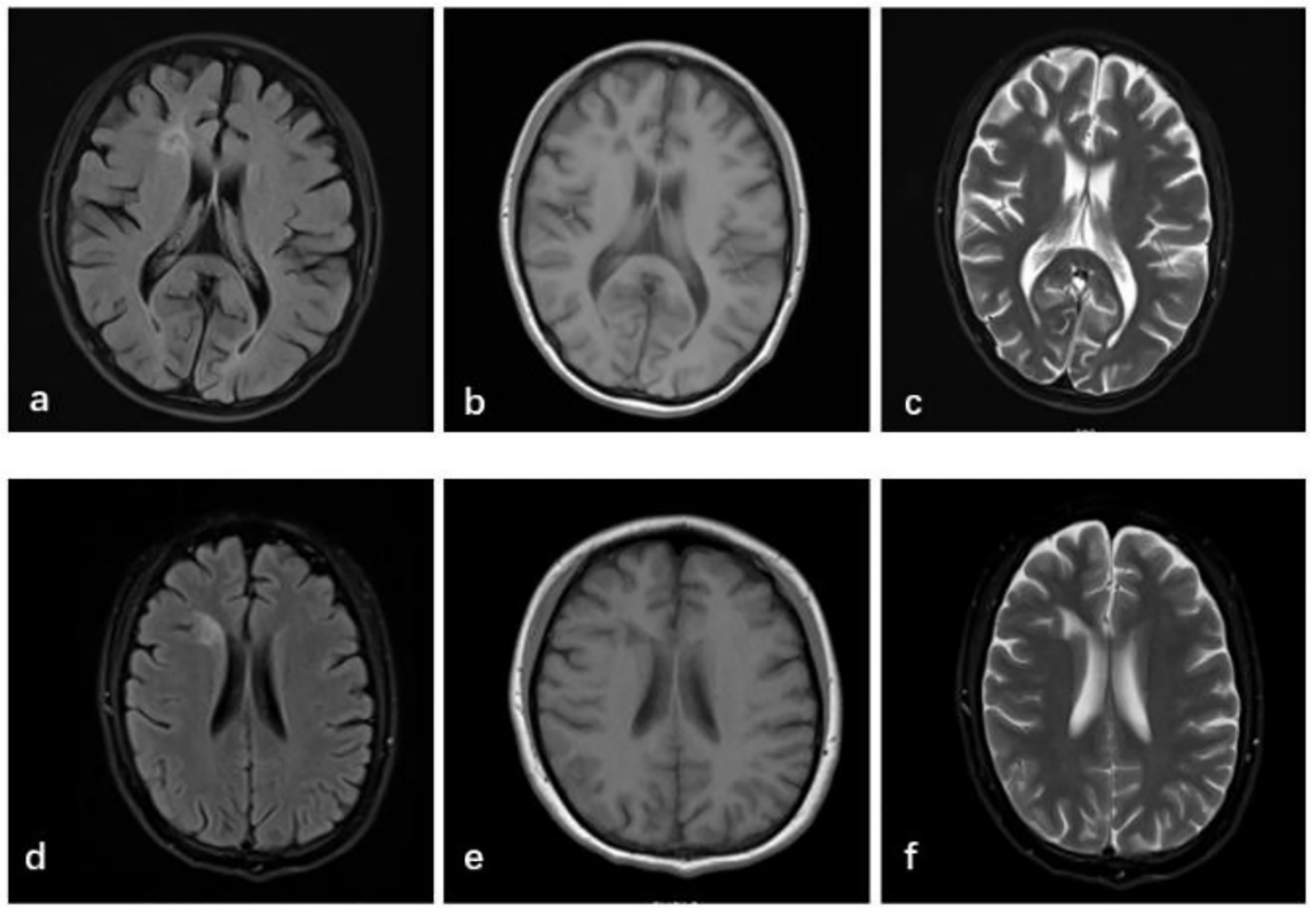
Brain MRI shows white matter lesions in the right anterior horn of the lateral ventricle, with slightly reduced FLAIR signal (a) and prolonged T1 (b) and T2 (c) signal intensities, suggesting focal encephalomalacia with gliosis or demyelination. These findings typically indicate changes after a brain injury, essentially representing that the brain tissue damage that had previous occurred has been resolved and is in a stable state. After 5 months, no new abnormalities were noted compared with prior imaging (d–f).
